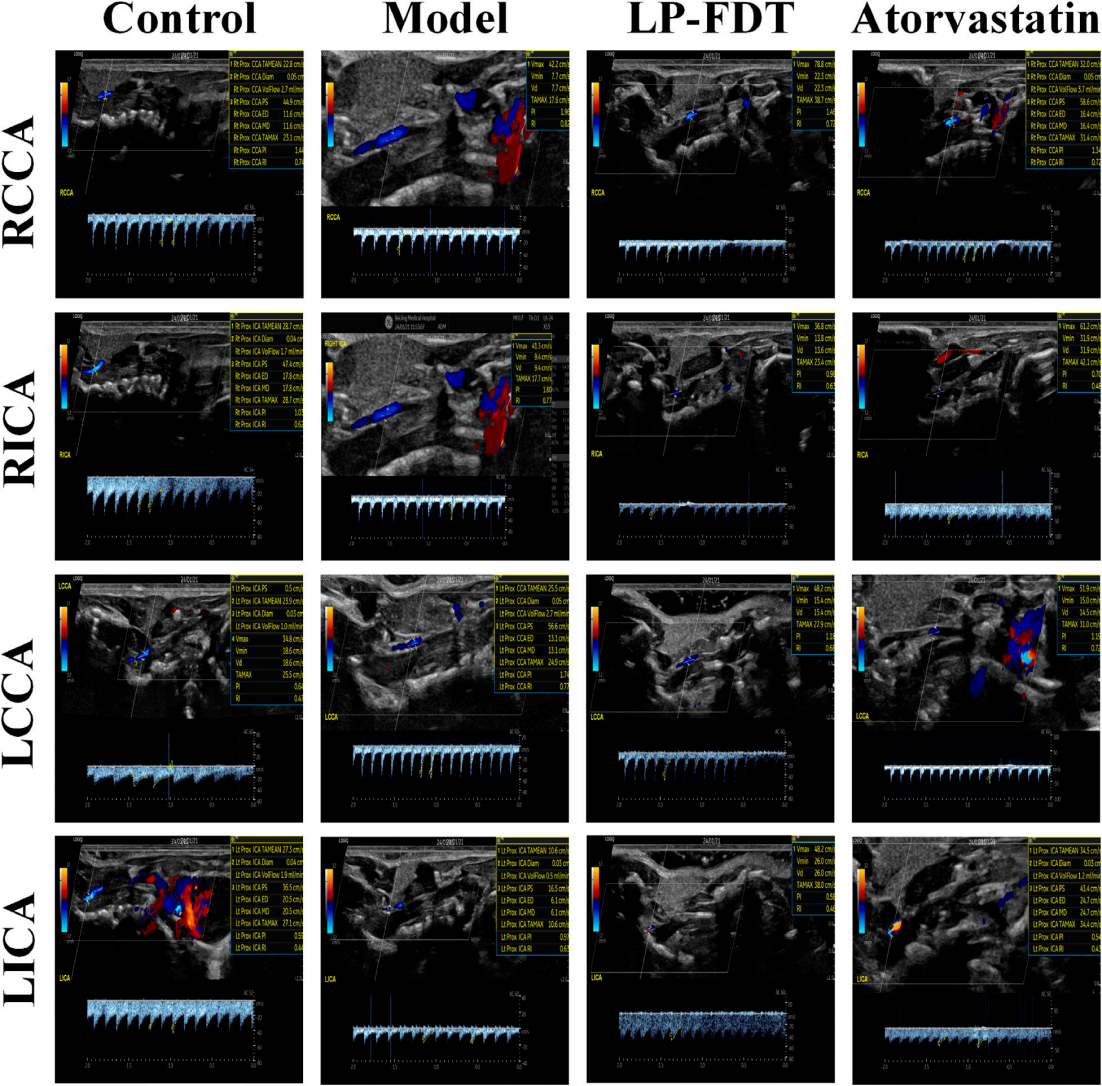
Blood flow velocity and blood flow related indexes of four carotid arteries (RCCA, RICA, LCCA, LICA) detected by ultrasound.
